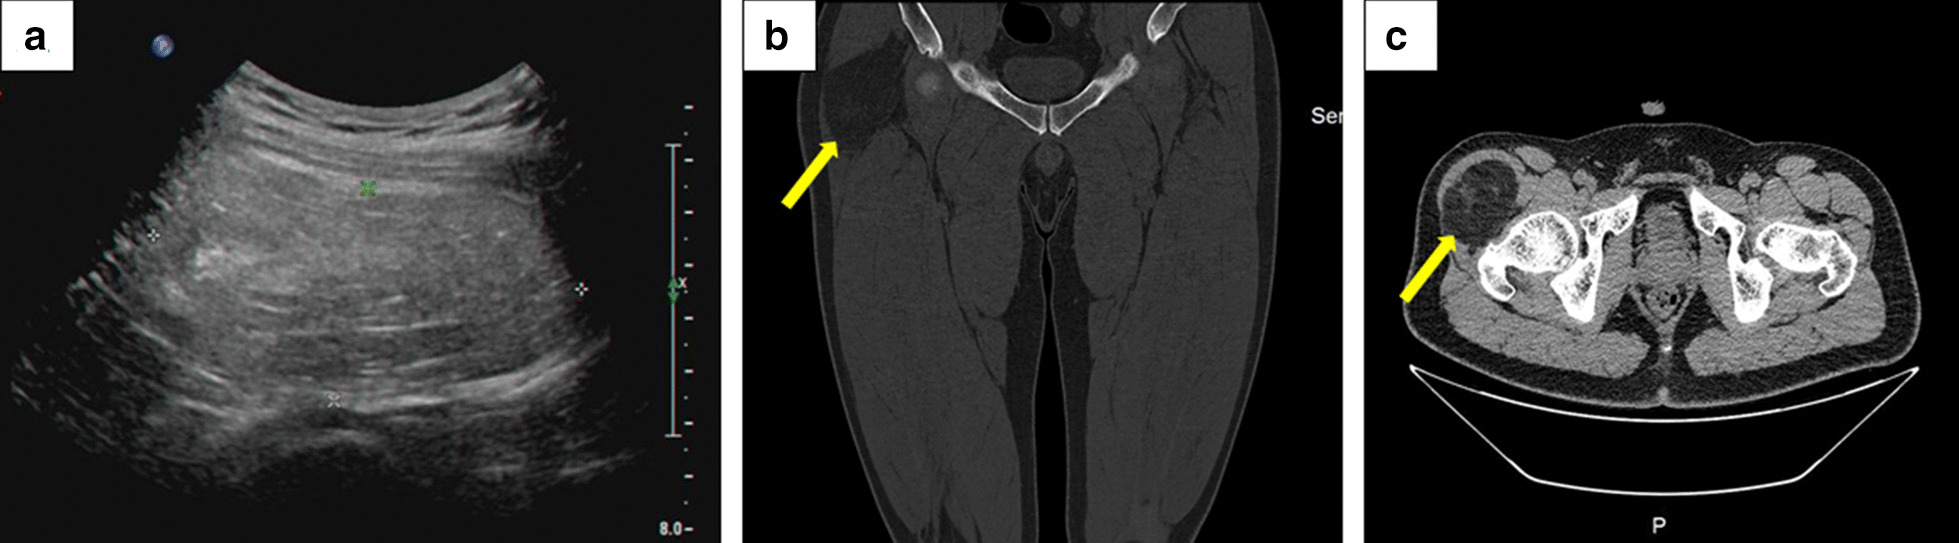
a Color Doppler ultrasound shows a near-isoechoic mass approximately 9.0 × 8.0 × 4.5 cm in size located in the muscular layer. The mass is irregularly shaped, with the edge of some parts showing a polygonal shape, and the boundary between the cortex and medulla is clear. b, c Computed tomography shows a massive low-density shadow in the medial side of the tensor fascia latae with a regular shape, a larger slice size of about 108 × 59 mm, and a CT value of about −61 HU. The adjacent muscle was compressed and deformed
